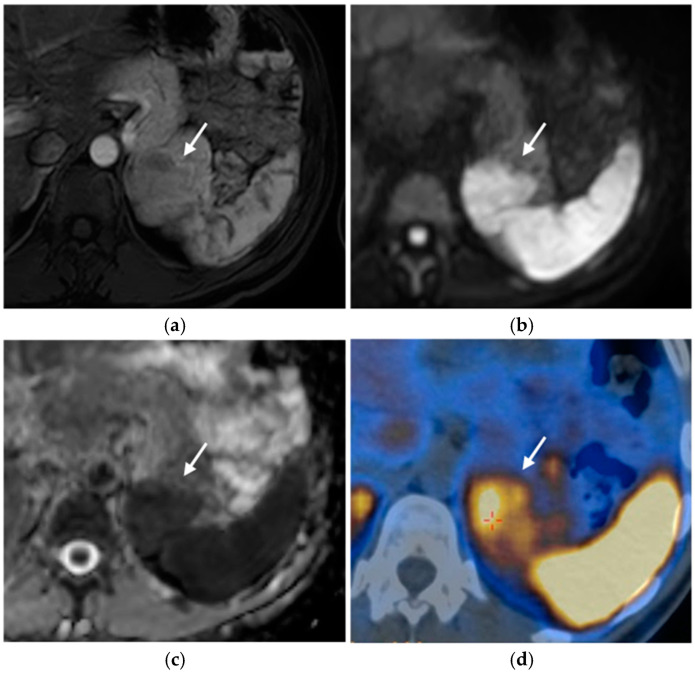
Insulinoma. Heterogeneously enhancing mass (arrows) in the pancreatic tail (a), with significant diffusion restriction (b,c) and avid uptake on PET-CT (d).
